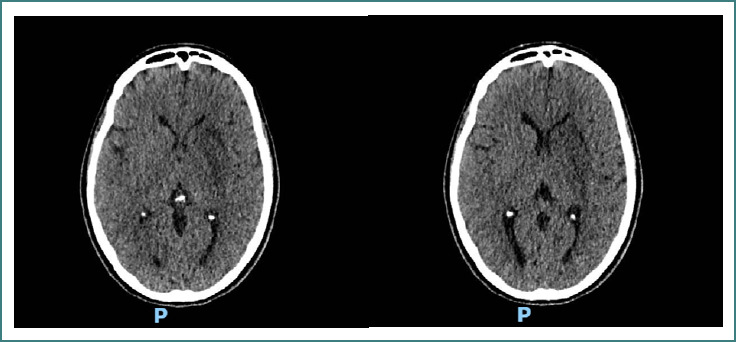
Brain CT scan at three-month follow-up
